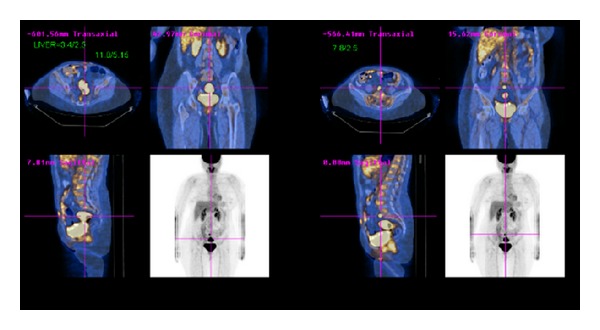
PET-CT showing a hypermetabolic rectosigmoid mass with multiple hypermetabolic enlarged lymph nodes.
